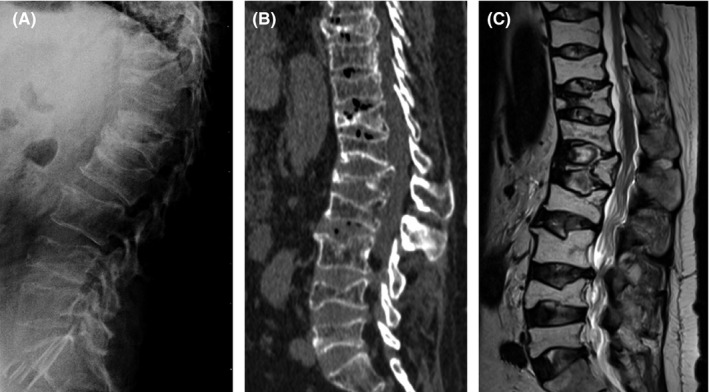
Initial imaging studies. (A) Lateral radiograph shows diffuse osteopenia and biconcave fracture of L1 and L4. (B) Sagittal CT image demonstrates additional compression fractures involving T12, T11, and T8. (C) Sagittal T2‐weighted MRI shows hyperintensity in body of L5, L4, L3, L2, L1, T12, T11, and T8 due to a compression fracture. The patient also had compression fracture at T5 (not shown)
